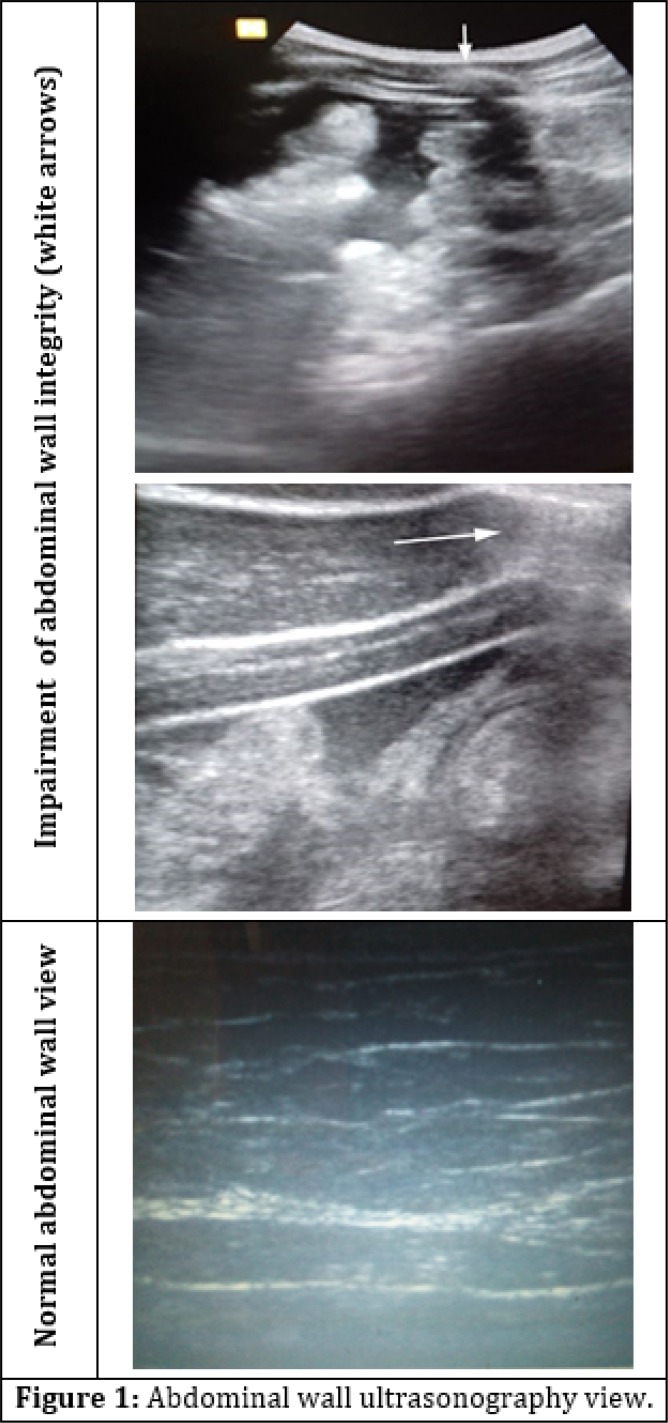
Abdominal wall ultrasonography view.
